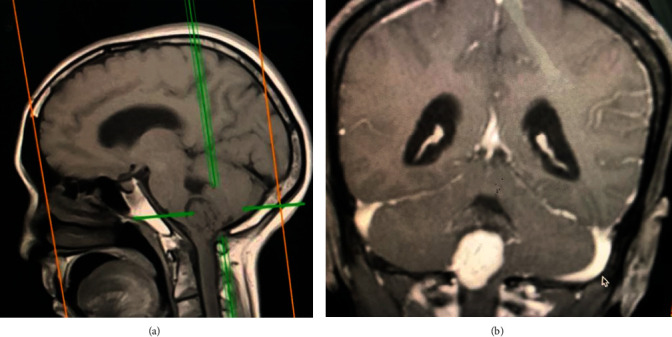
Sagittal T1 (a) and coronal T2 (b) MRI sequences showing a well-defined mass lesion measuring 2.2^∗^2.0 cm at the region of cerebellomedullary cistern.
